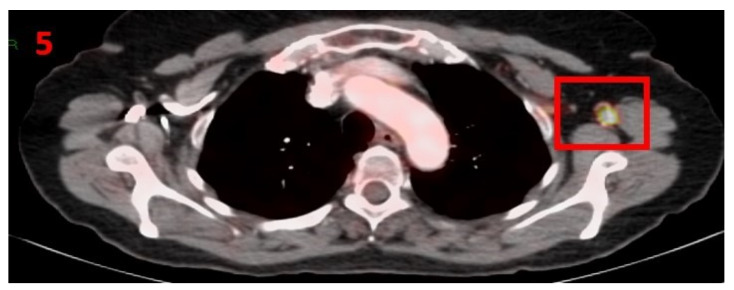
[18F]FDG PET-CT figure showing hypermetabolic uptake in the 5th patient with lymphadenopathy in the left axillary region (red square).
